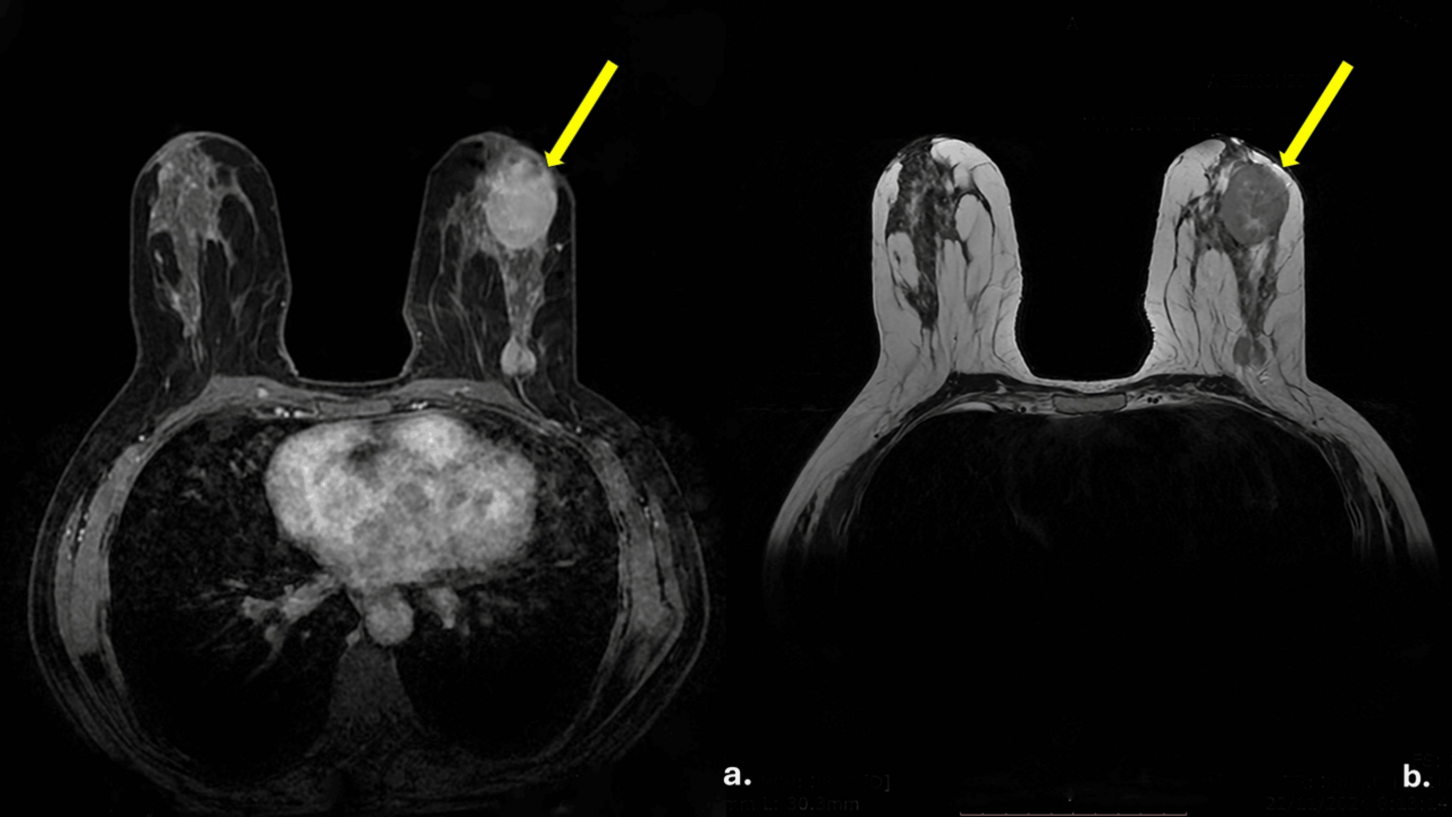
Bilateral breast MRI images The images demonstrate a large, irregular lesion (measuring 3,9 x 3,1cm) in the UOQ of the left breast (yellow arrows). (a) Axial T1-weighted post-contrast fat-suppressed image (3D GRE) shows intense enhancement of the dominant lesion (b) Axial T2-weighted fat-suppressed (STIR) image shows the same lesion as hyperintense. These findings reflect features of the BI-RADS IVa classification. UOQ: Upper outer quadrant, MRI: Magnetic resonance imaging.
